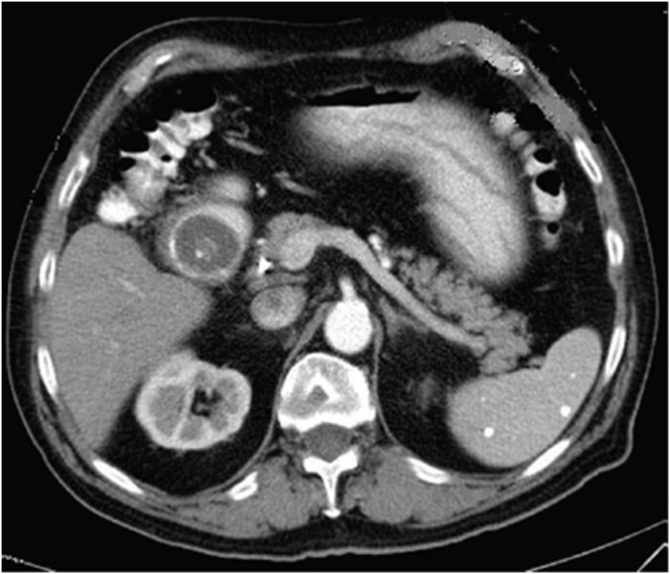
Abdominal CT scan showing a subtotal stenosis of the lumen of the second duodenal portion.
